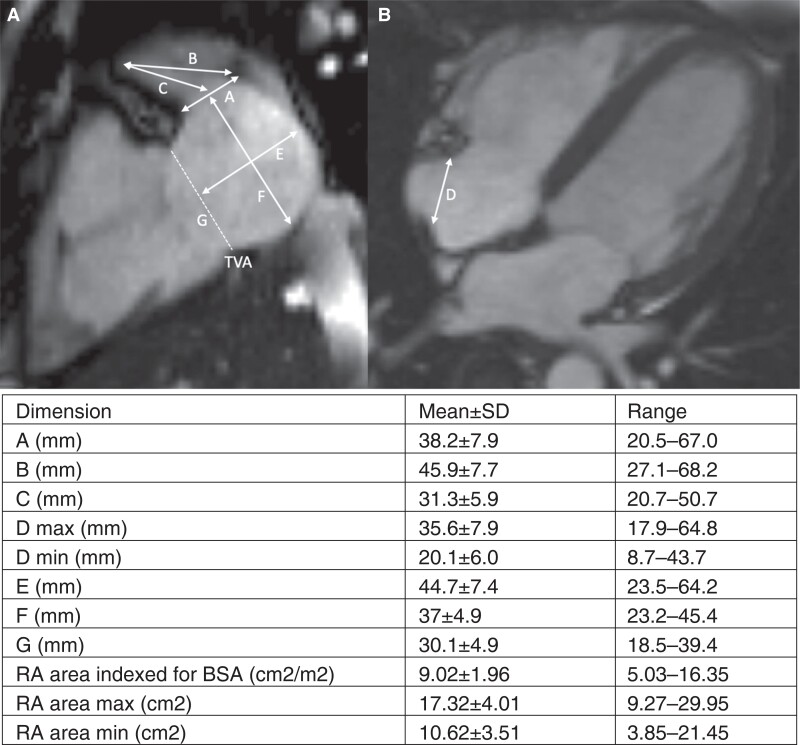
Cardiac MRI dimensions analysed. Representative MRI images demonstrating the measurements taken and the resultant mean measurement; (*A*) two-chamber view. (B) Four-chamber view. Measurements noted: A—RAA ostium, B—RAA depth to CT, C—RAA depth to mid-point of RAA ostium, D—CT to lateral TVA, E—RA diameter (2Ch view) perpendicular to TV annulus, F—RA diameter (2Ch view) perpendicular to RAA ostium, G—TVA diameter (2Ch view). BSA, body surface area; CT, crista terminalis; MRI, magnetic resonance imaging; RA, right atrium; RAA, right atrial appendage; TVA, tricuspid valve annulus.
